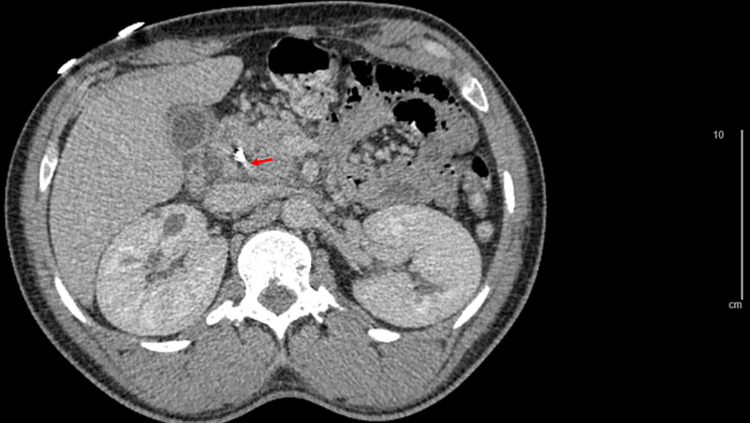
CT scan showing area of contrast blush concerning pseudoaneurysm (red arrow), and pancreatitis with peripancreatic free fluid collection at 1.42x magnification.
